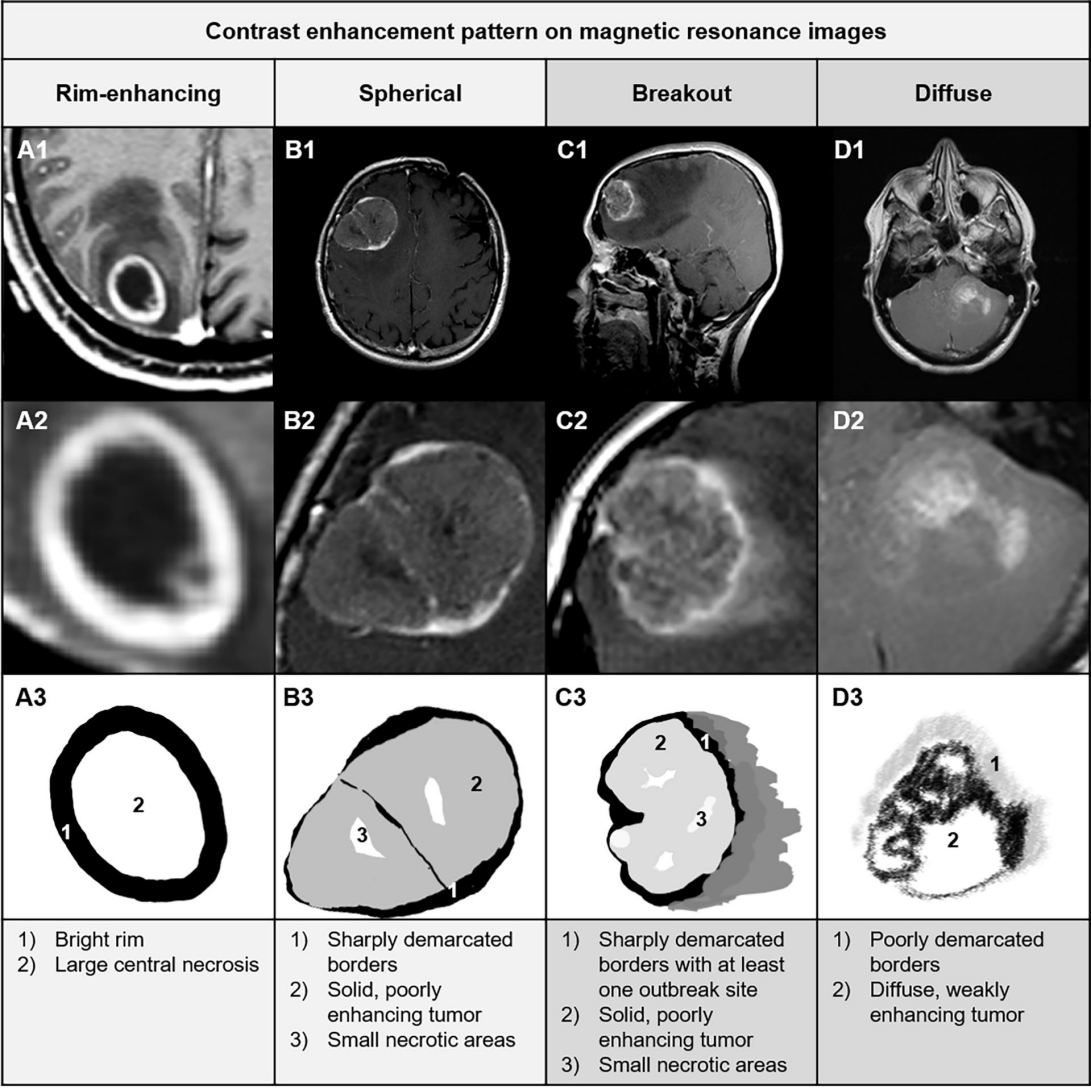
Definition, examples (A1–D1) and description (A2–D2) of the four different MRI subtypes: (A) rim-enhancing, (B) spherical, (C) breakout, and (D) diffuse.
